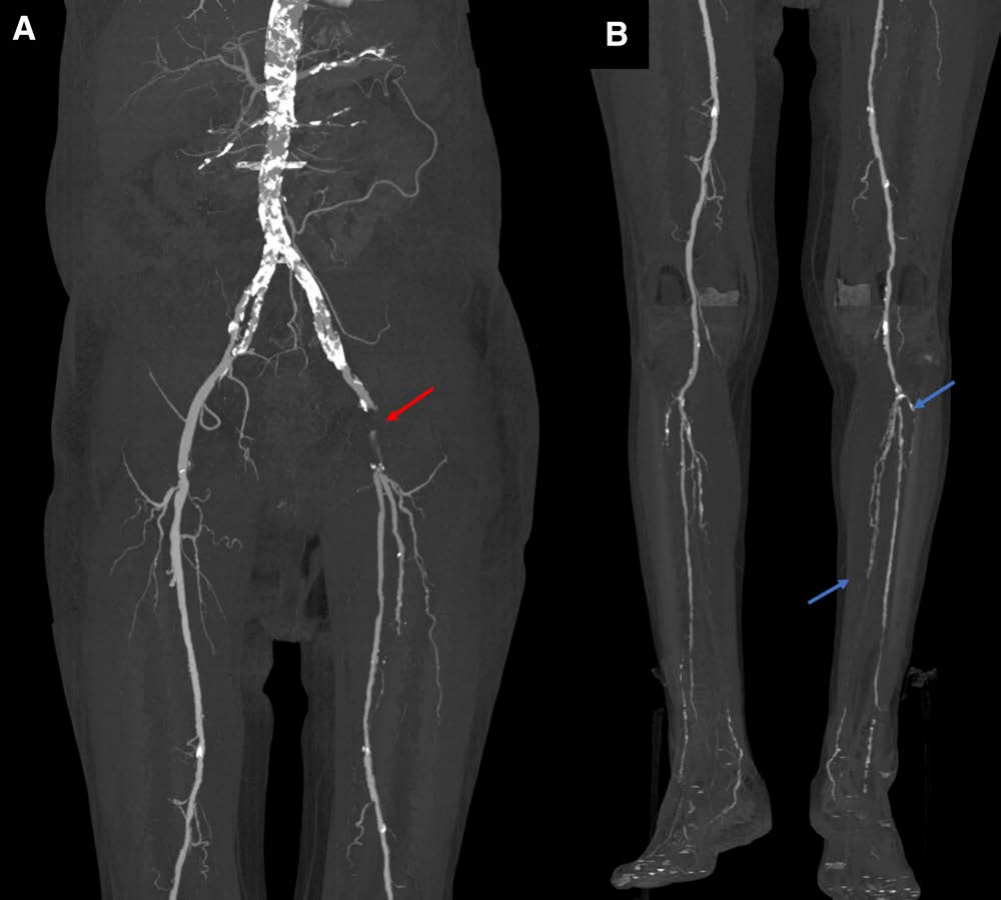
Computed tomography angiography of the lower extremities conducted 14 days after the decompressive laminectomy revealing a left common femoral artery occlusion (red arrow) and multifocal severe stenoses at the bilateral anterior and posterior tibial arteries (blue arrows).
